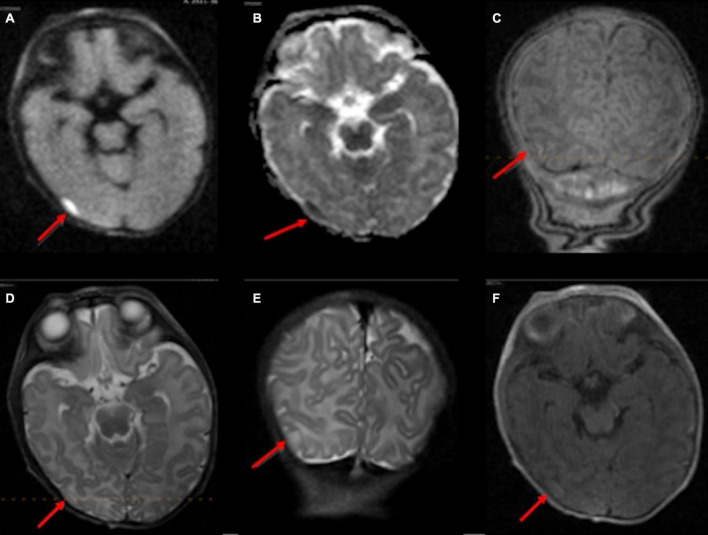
In an 11-day-old term neonate with a history of decreased respiratory drive, the 1-Tesla point-of-care MRI showed a subdural collection overlying the right temporo-occipital junction with reduced diffusion on DWI (A) and ADC (B), which appeared isointense on T1 (C,F) and T2 (D,E). No other brain imaging was performed.
